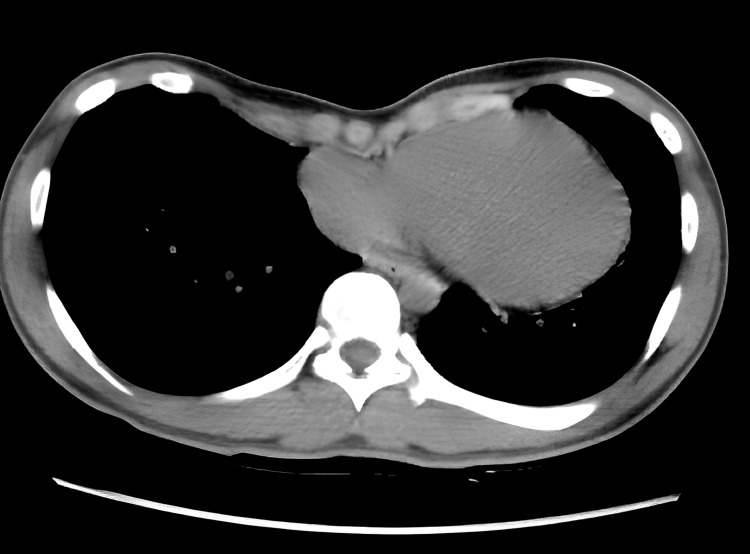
Chest CT image of Case 2 (Haller index=5.9) Symmetrical depression of the sternum and mild cardiac compression are observed.
